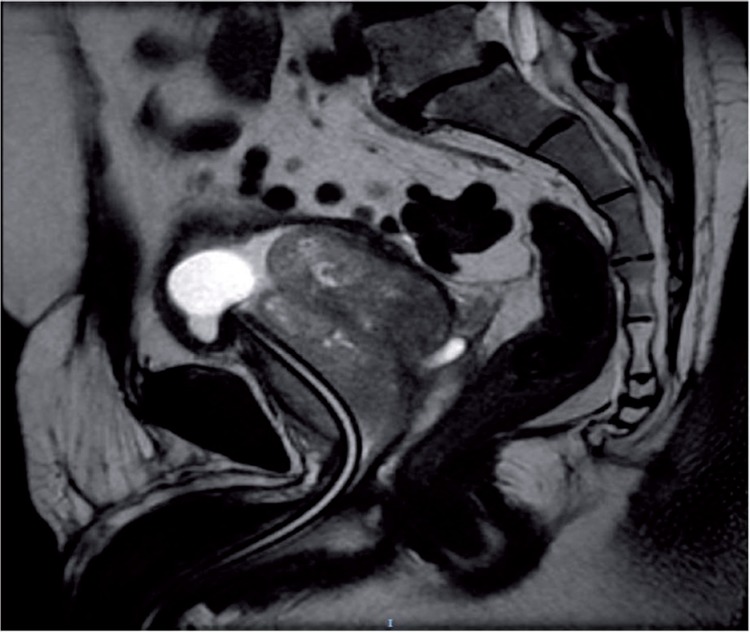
T2-weighted prostate MRI in sagittal view showing a diffusely enlarged prostate. Note also the indwelling bladder catheter displaced anteriorly.
